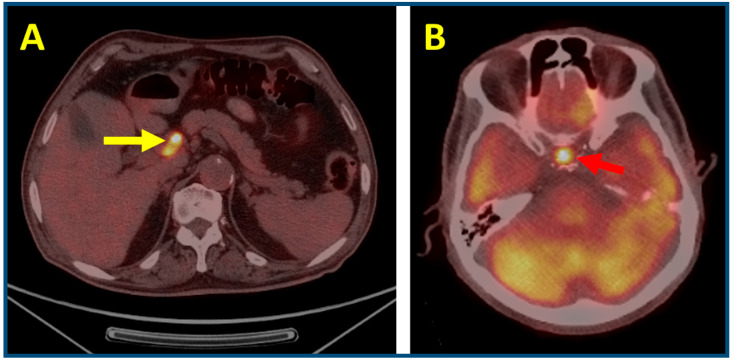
A 65-year-old man with melanoma metastatic to abdominal lymph nodes ((A); axial PET/CT fusion images, yellow arrow) underwent a combination of ipilimumab and nivolumab. Following treatment initiation, an ^18^F-FDG PET/CT scan was performed to assess response. A focal pituitary uptake, highly suspicious for hypophysitis, was detected ((B); axial PET/CT fusion images, red arrow). Although the patient was asymptomatic, biochemical evaluation confirmed panhypopituitarism, and appropriate endocrine therapy was started.
